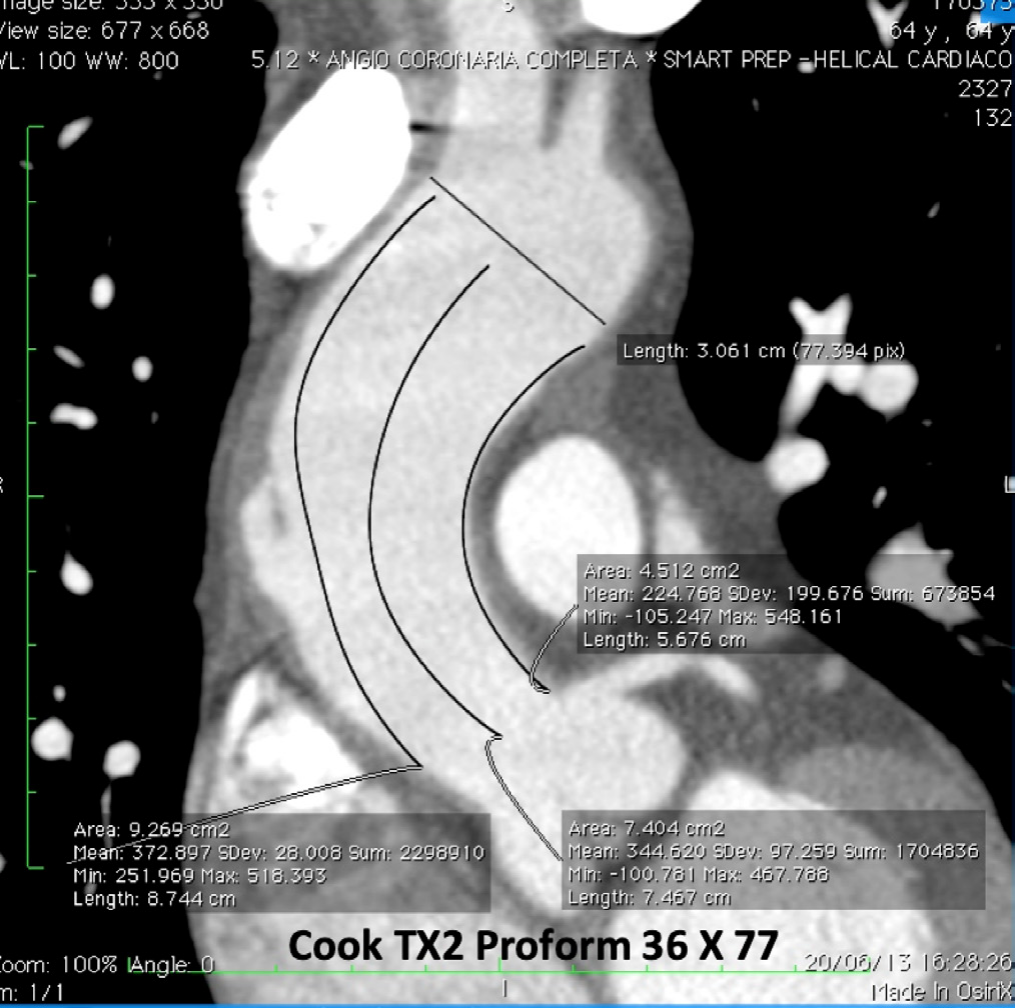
Ascending aortic dissection. Planning endovascular treatment with preoperative contrast-enhanced computed tomography.
